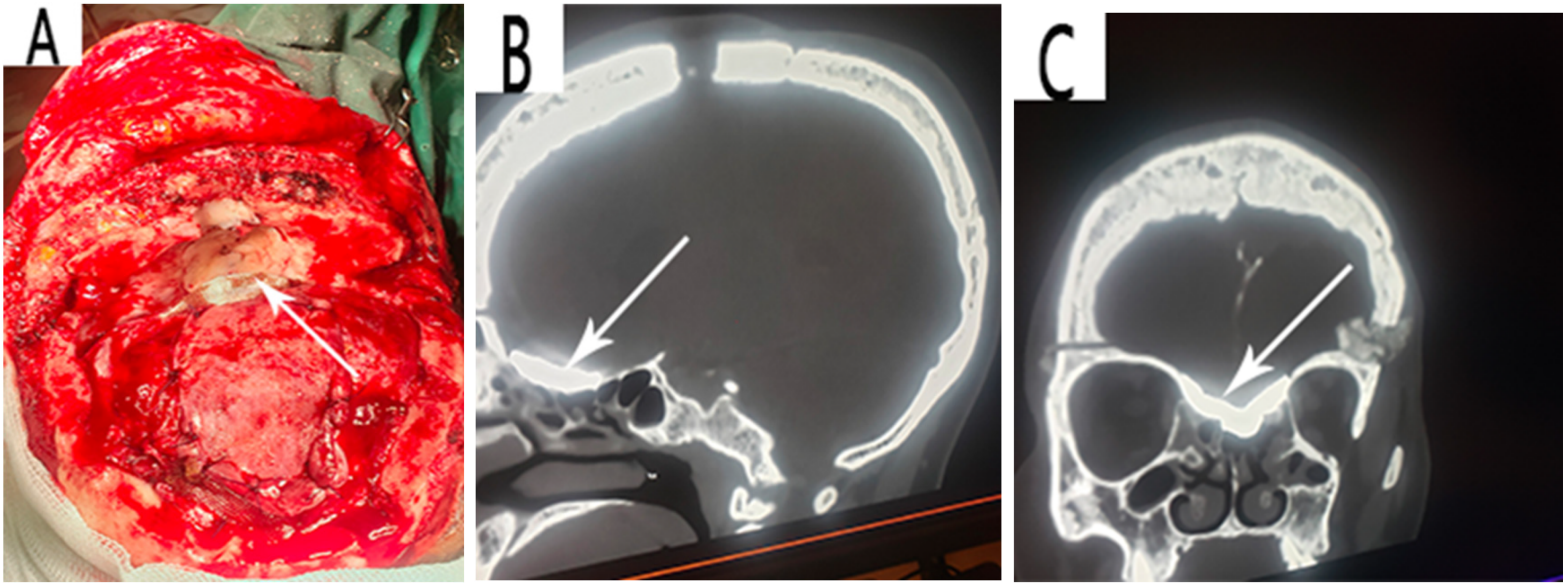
(A) Reconstruction of the defects with bis-GMA and pericranial flap. A follow-up CT scan showing visible fusion of the bis-GMA with the surrounding bone and complete sealing of the skull base bony defect (B, C).
